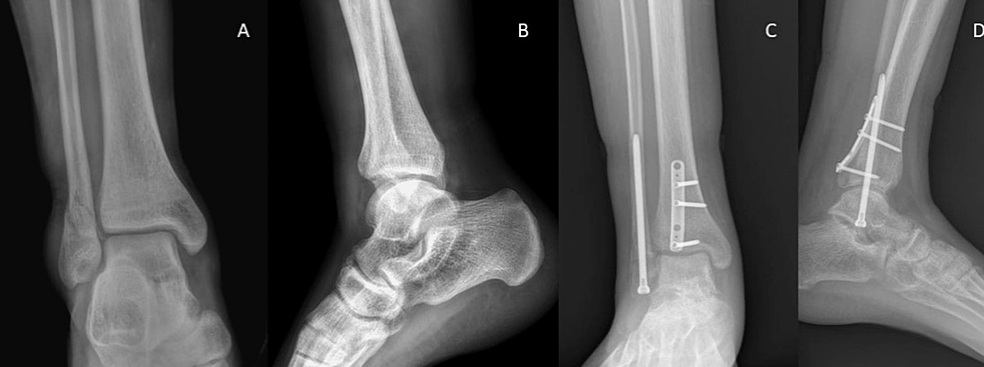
Screw fixation (A) Preoperative anteroposterior radiograph, (B) preoperative lateral radiograph, (C) postoperative (one year follow-up) anteroposterior radiograph, (D) postoperative (one year follow-up) lateral radiograph
